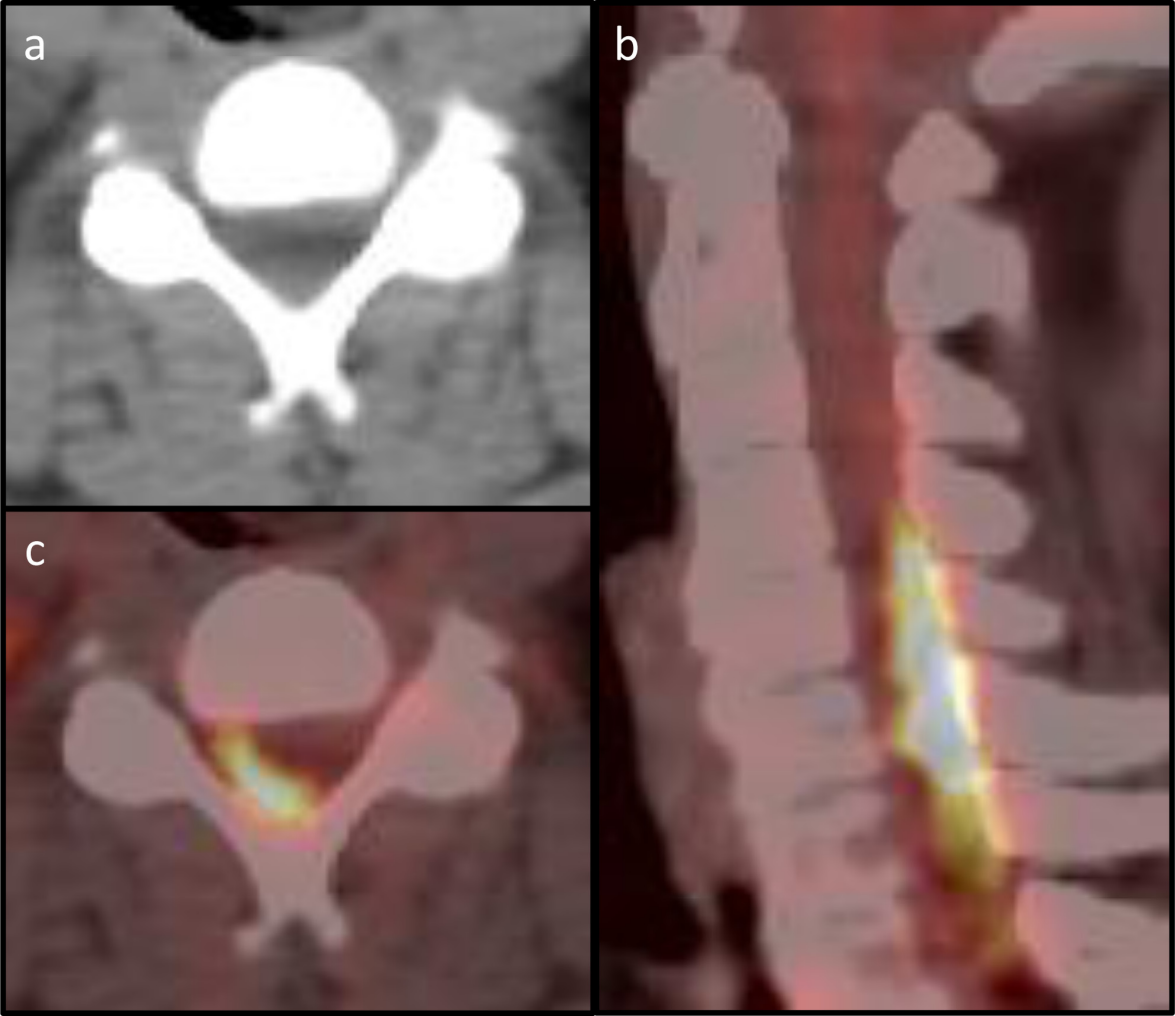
PET-CT of the cervical spine. (a) Axial CT at vertebral level C4 with barely visible posterior epidural mass. (b) Axial PET-CT of vertebral level C4 with notable FDG-avid disease in the spinal canal. (c) Sagittal PET-CT with FDG-avid disease posteriorly within the spinal canal.
